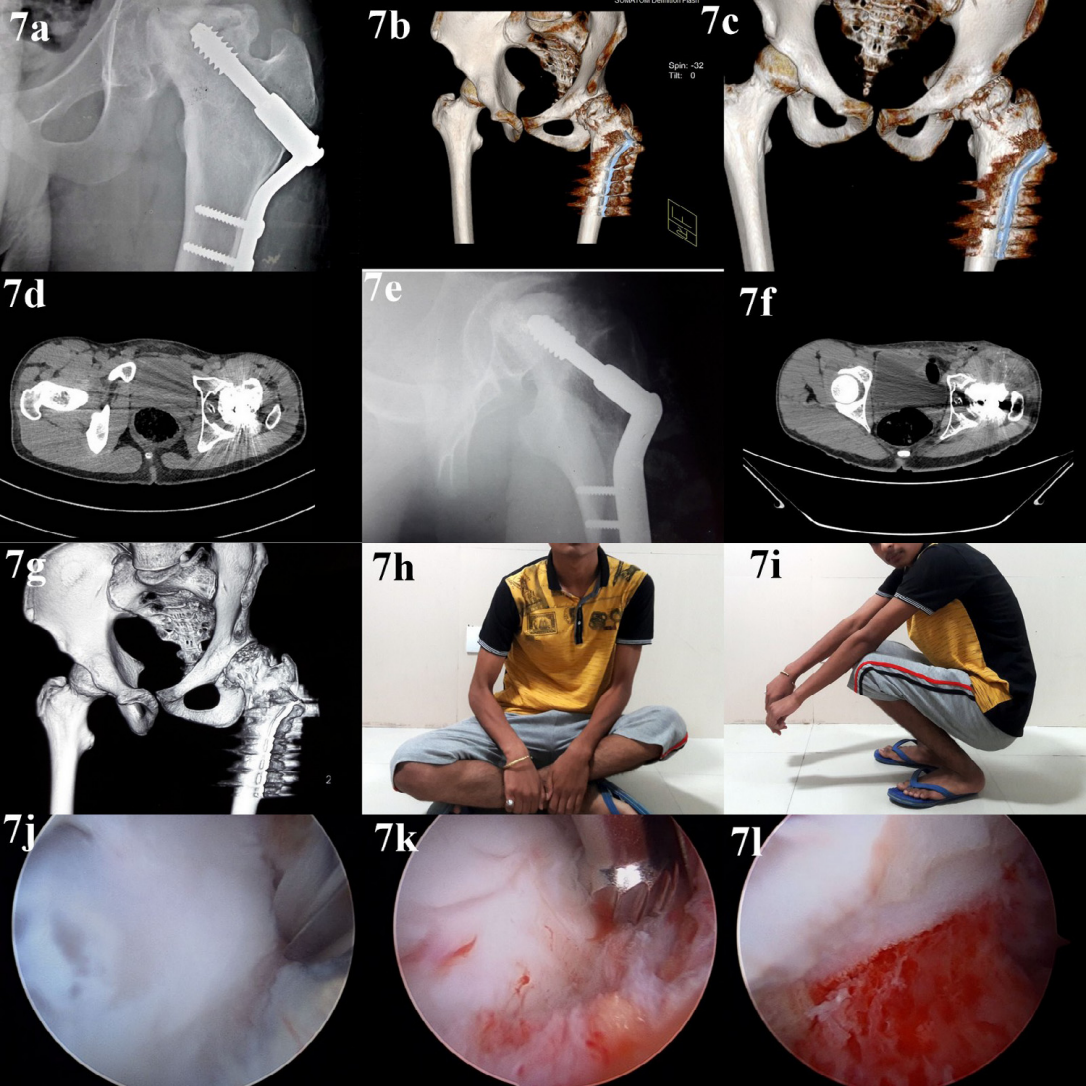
Follow-up of a 22-year-old male presenting with symptoms of hip impingement after post-traumatic ONFH. The patient initially sustained neck of femur fracture, which was treated with closed reduction and internal fixation with cannulated cancellous screws. The fracture failed to unite at 6 months and valgus osteotomy of proximal femur with internal fixation with a double-angle dynamic hip screw was carried out. The fracture united and patient was asymptomatic for 1 year. Subsequently, he presented with hip pain and radiograph showed collapse of femoral head indicating ONFH. He was managed conservatively for 1.5 years. He then developed symptoms of hip impingement, with restriction of hip flexion and rotations. There was no pain on weight bearing or walking. He was managed with arthroscopic osteochondroplasty: panel a: preoperative radiograph showing cam deformity in right femoral head; panels b–d: preoperative CT scan showing extent and location of cam deformity in the femoral head; panel e: post-operative radiograph showing restoration of femoral head sphericity; panels f and g: post-operative CT scan after removal of cam deformity; panels h and i: post-operative follow-up images showing restoration of hip range of motion; panel j: intraoperative image of hip arthroscopy showing identification of the cam deformity; panel k: intraoperative image of hip arthroscopy showing resection of the cam deformity; panel l: intraoperative image of hip arthroscopy after removal of the deformity.
